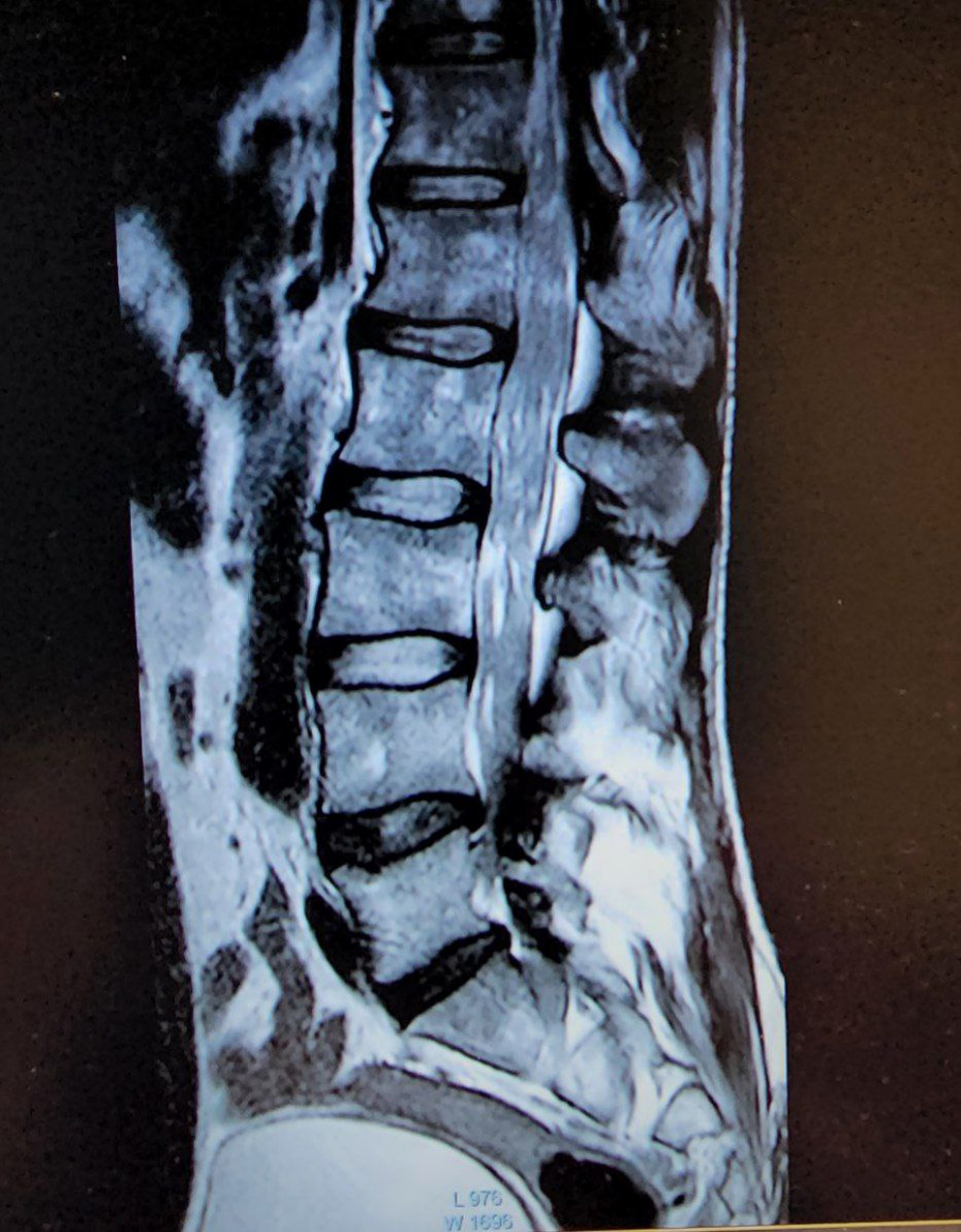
MRI of the lesion in the dorsal and lumbar columns of the spinal cord (T2).
